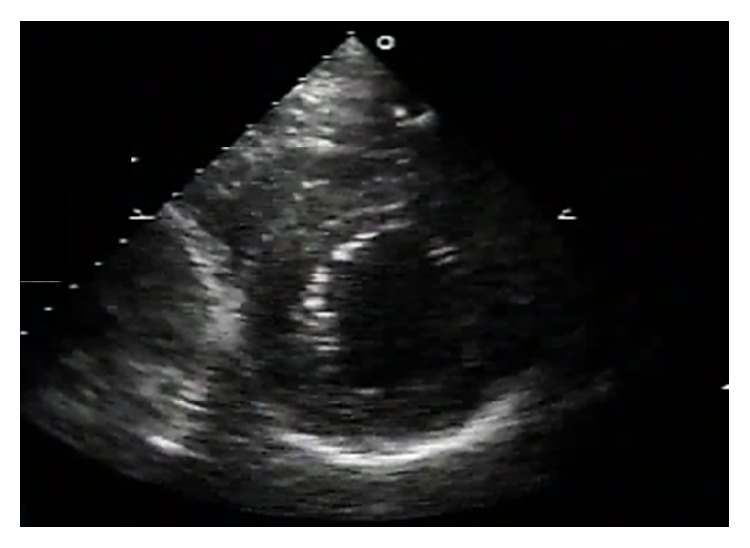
Echocardiography at 6-week follow-up demonstrating the resolution of the hematoma.
